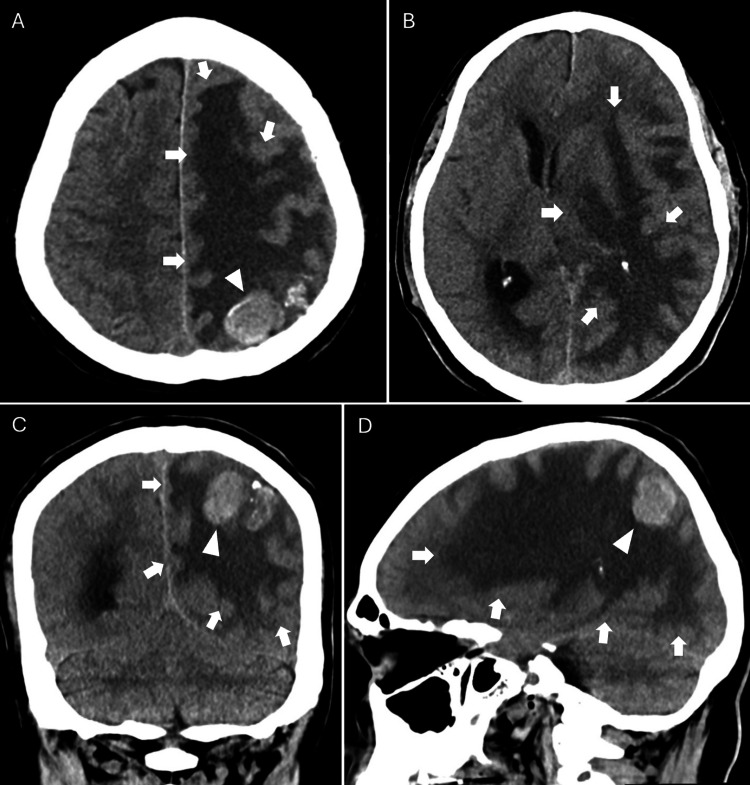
Cranial CT obtained 15 years after stereotactic radiosurgery CT: computed tomography (A-B) Axial, (C) coronal, and (D) sagittal views demonstrate a hyperdense, round lesion (arrowheads) at the cortical surface of the left parietal lobe. Extensive, frond-like hypodensity consistent with progressive vasogenic edema (arrows) is observed, involving the left frontal, parietal, and occipital lobes, accompanied by a rightward midline shift
